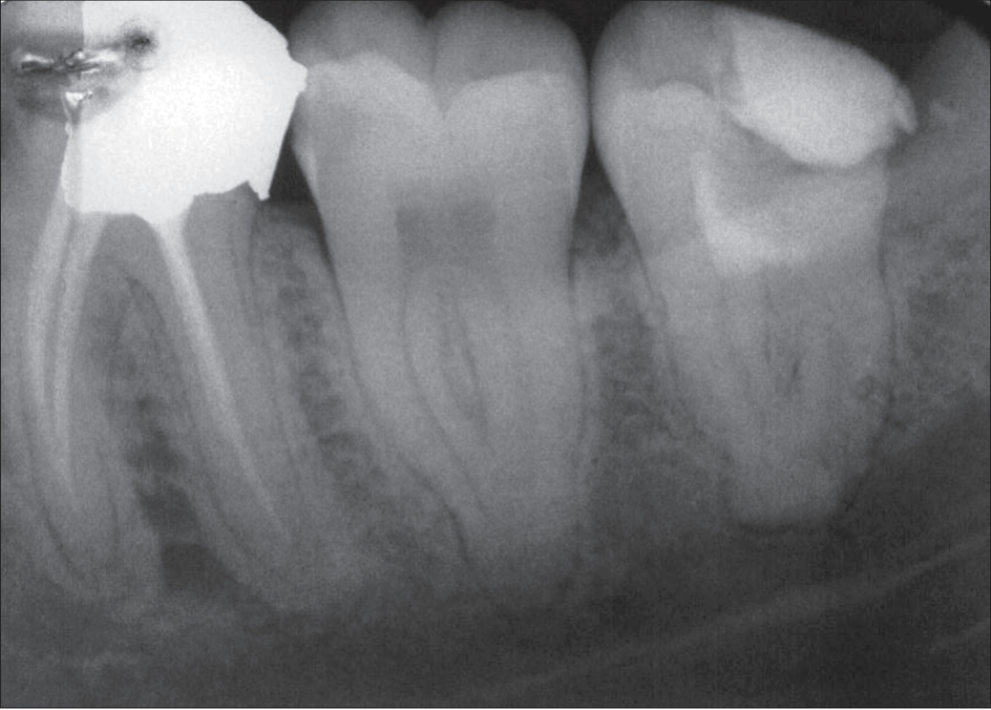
Postoperative radiograph showing pulpotomy with new endodontic material
